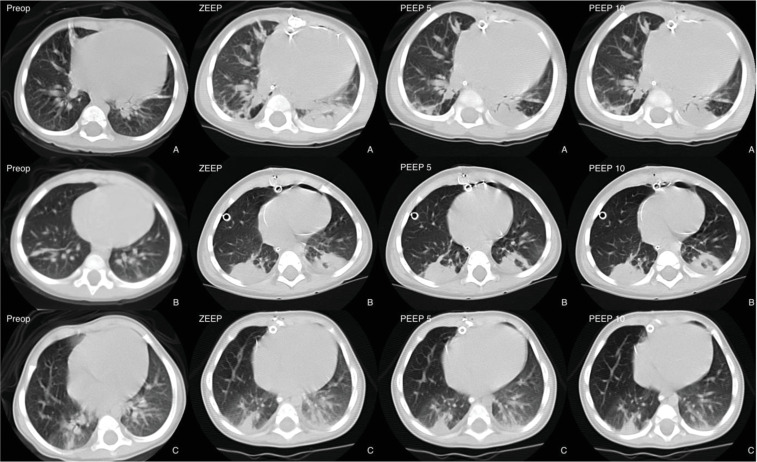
Representative CT images obtained 1 cm above the diaphragm in the preoperative (Preop) and postoperative periods in zero PEEP conditions (zero PEEP), 5 cm H_2_O PEEP (PEEP 5) and 10 cm H_2_O PEEP (PEEP 10) in three patients (A, B and C).
